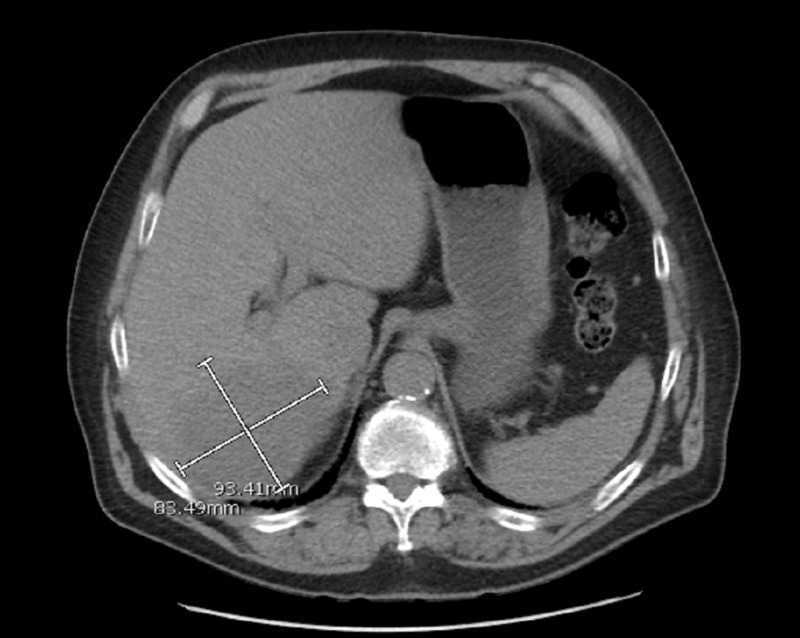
CT abdomen/pelvis (axial view) showing a hypodense mass in the right posterior lobe measuring 9.3 x 8.3 cm in size (see marked scale)
